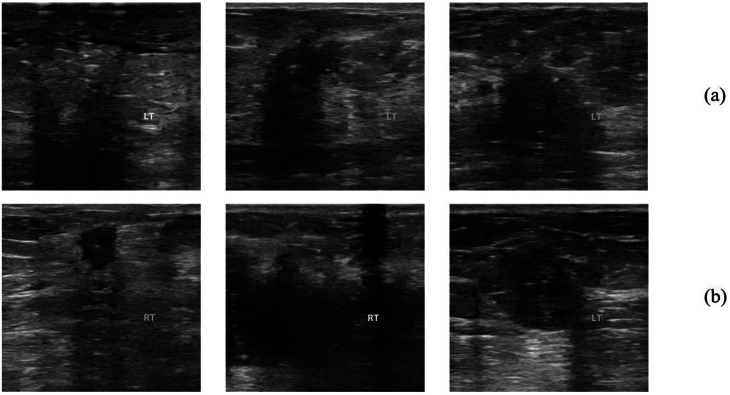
(a) Involved and (b) noninvolved axillary lymph node ultrasound images from the dataset
